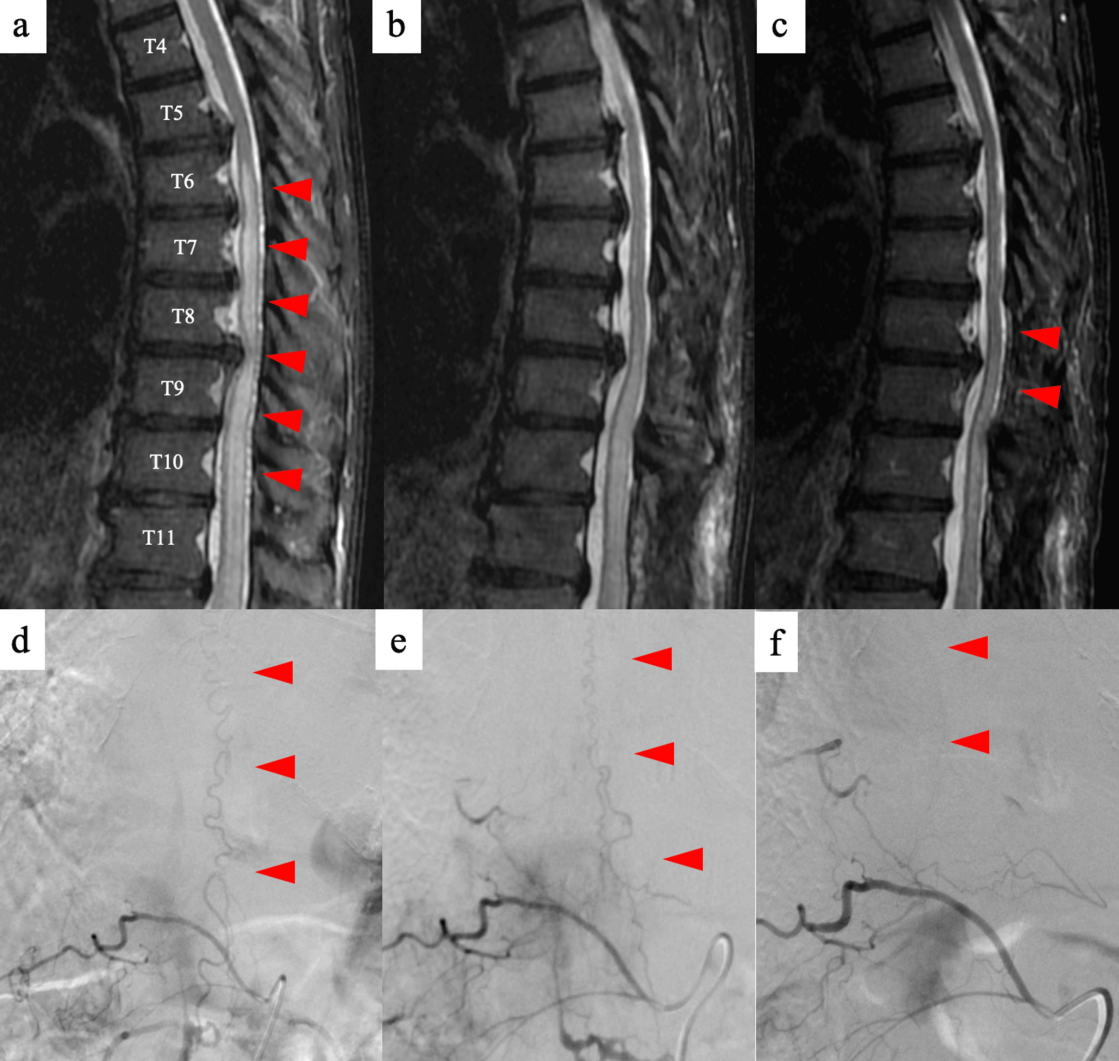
Imaging findings before and after surgery, and after embolization in Case 2 (a) T2-weighted MRI shows hyperintense areas within the spinal cord at and below the T6/7 level, with flow voids noted along the dorsal aspect of the cord from T8 to T11. Spinal cord compression is observed at the T5/6, T6/7, T7/8, and T8/9 intervertebral levels, with particularly severe stenosis noted at the T8/9 level; (b) Postoperative T2-weighted MRI; (c) T2-weighted MRI scan after embolization (nine months after surgery) shows persistent high signal intensity within the spinal cord; (d) Angiography before surgery shows an sdAVF with T11-12 right root artery as the feeding vessel; (e) Angiography after surgery shows a persisting spinal arterio-venous fistula fed by the right radicular artery (dorsal somatic branch) of right T11 intercostal; (f) Angiography after embolization (nine months after surgery) shows a marked reduction in abnormal vasculature, although minimal residual shunting remains. The red arrowheads indicate findings of a spinal dural arteriovenous fistula.
